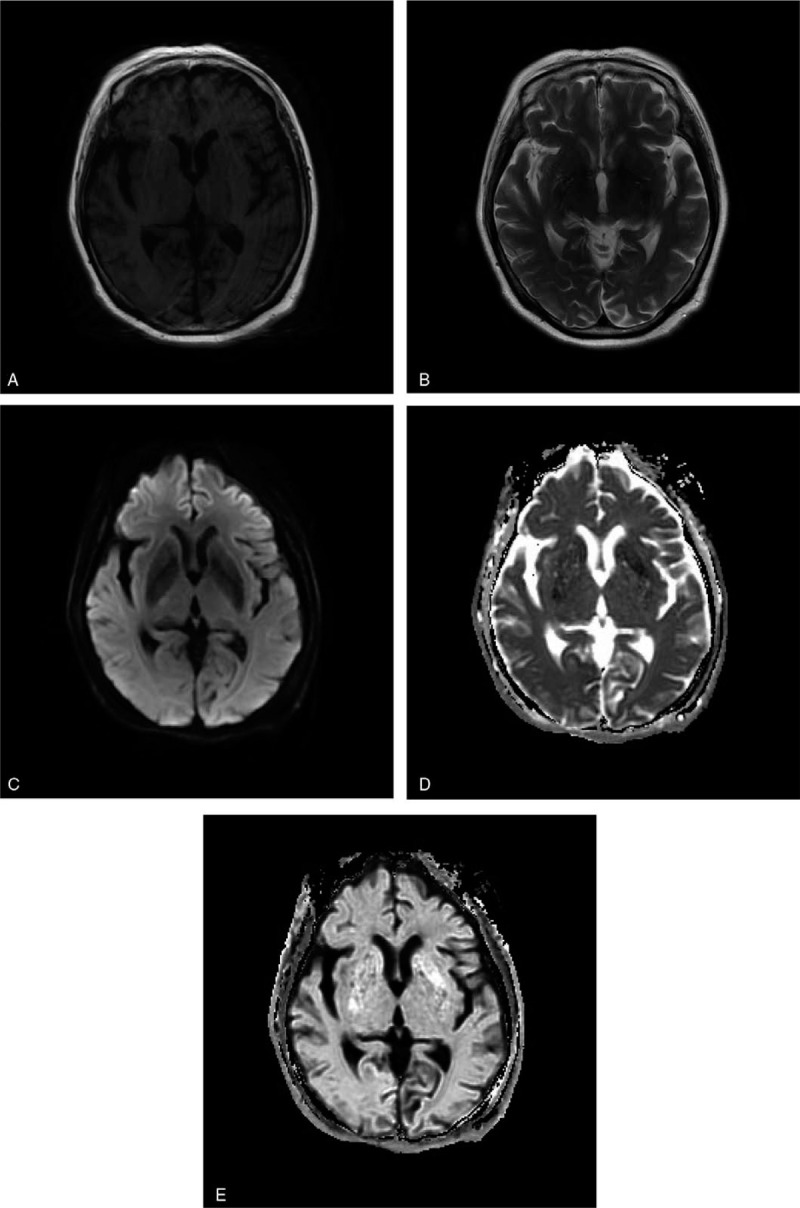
Brain magnetic resonance image (MRI) on the second day of the symptom: T1-MRI(2A), T2-MRI(2B), and DWI(2C) were normal, ADC(2D) showed low signals in the left basal ganglia, and high signals in E-ADC(2E) in the same part.
